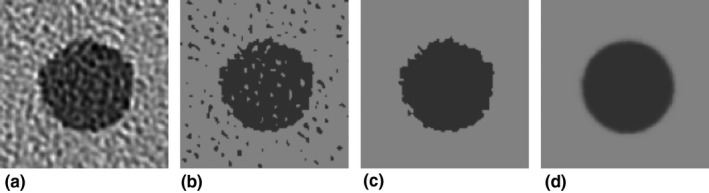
(a) An example of a computed tomography image of cylindrical polystyrene (PS) object reconstructed using the IMR *Body SharpPlus* (level 1) algorithm. (b) Binary image obtained from the image in (a) by thresholding. (c) Effective object function obtained from the image in (b). (d) The mean image obtained by averaging all of the 200 effective object functions. The window settings are WL/WW = 25/450 HU.
